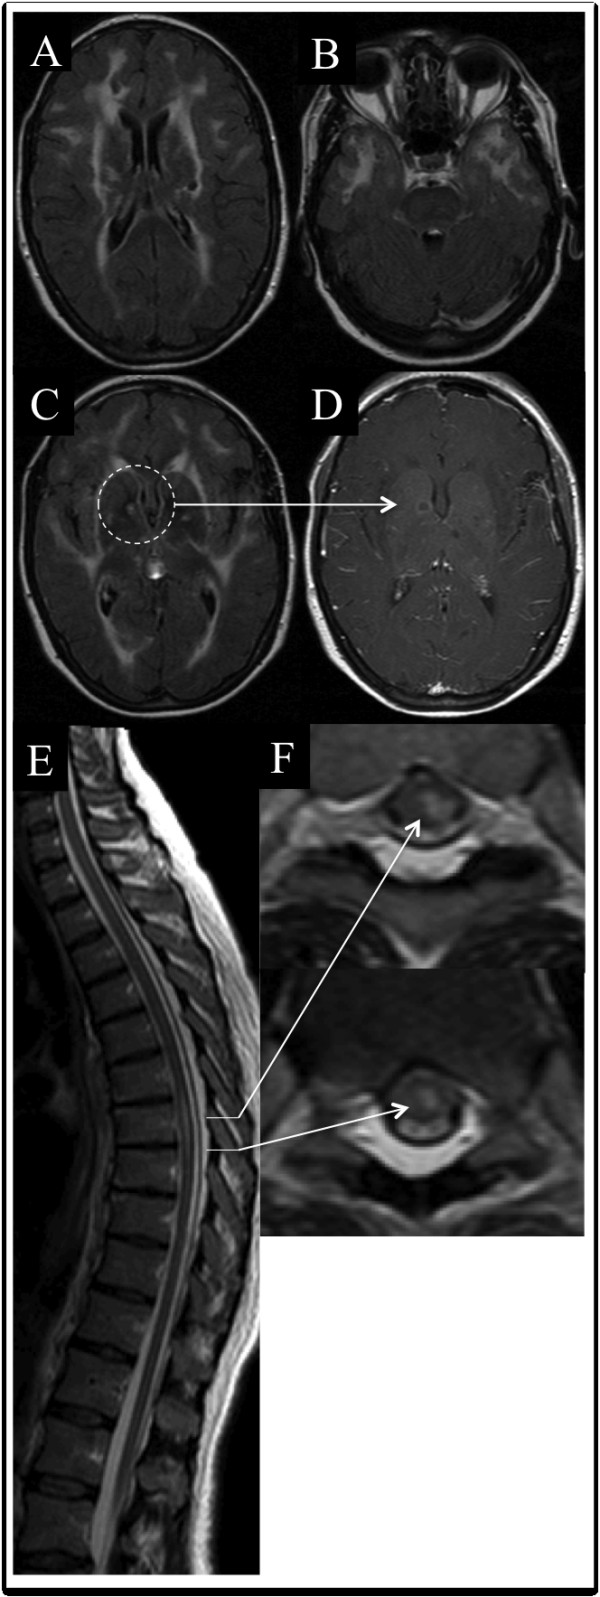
Brain and spinal cord MRI of patient 1. Flair sequence showing brain abnormalities typical of CADASIL, involving the external capsule, typical lacunar infarcts (A), and temporal lobes (B). Lesion in the right internal capsule (C) were enhanced by gadolinium injection on the T1-weighted image (D, arrow). A thoracic spinal cord lesion is noted on the longitudinal T2-weighted image (E), corresponding to partial myelitis in the transverse plane (F).
